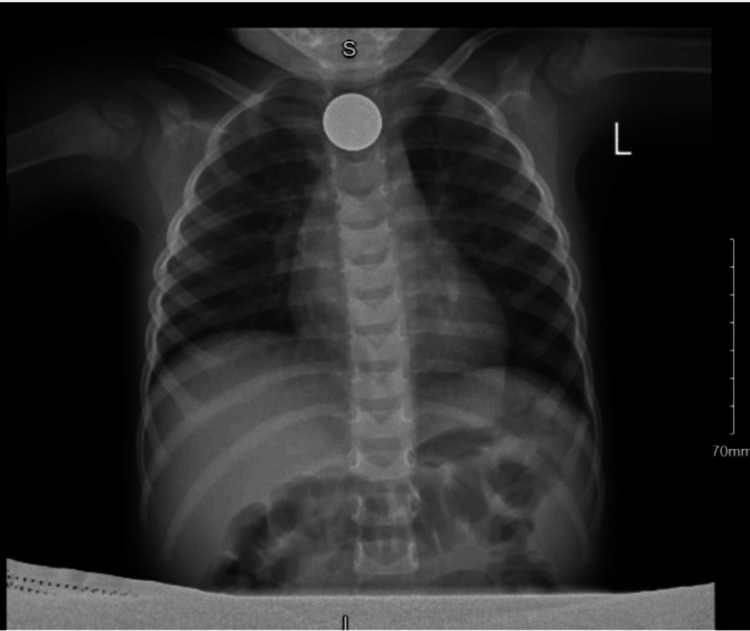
Preoperative chest X-ray demonstrating a round radiopaque foreign body, consistent with a coin, lodged in the upper esophagus.
